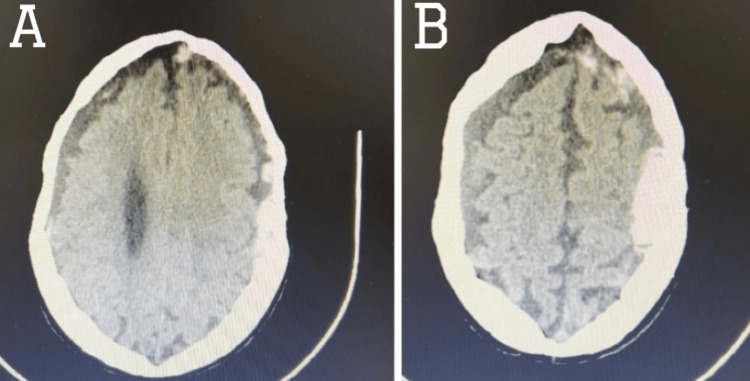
Axial cut of non-contrast-enhanced brain CT Axial cut of non-contrast CT scan of the brain showing bilateral subdural hematomas of different ages with (A) acute component on the left frontoparietal region with minimal mass effect and (B) associated minimal subarachnoid hemorrhage.
